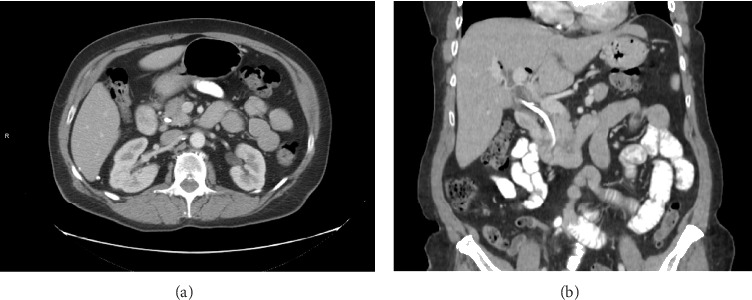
CT abdomen and pelvis. Complete resolution of pancreatic head fullness on the axial image (a) and the coronal image with a CBD stent in place (b).
